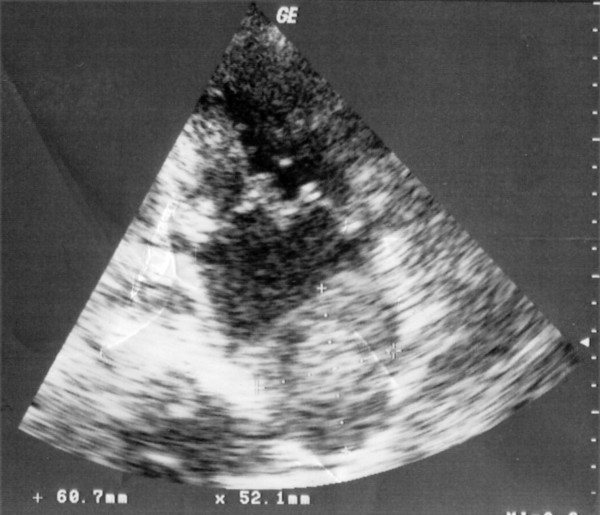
Preoperative echocardiography. Echocardiographic confirmation of the large mass in the ventricle of left atrium.
